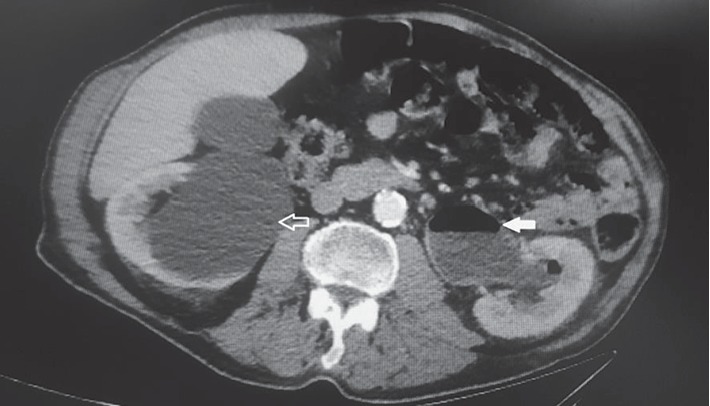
Computerized tomographic (CT) scan of the abdomen and pelvis. Left hydronephrosis with presence of air in the collecting system suggestive of left-sided emphysematous pyelonephritis (arrow). Right parapelvic cyst (empty arrow).
